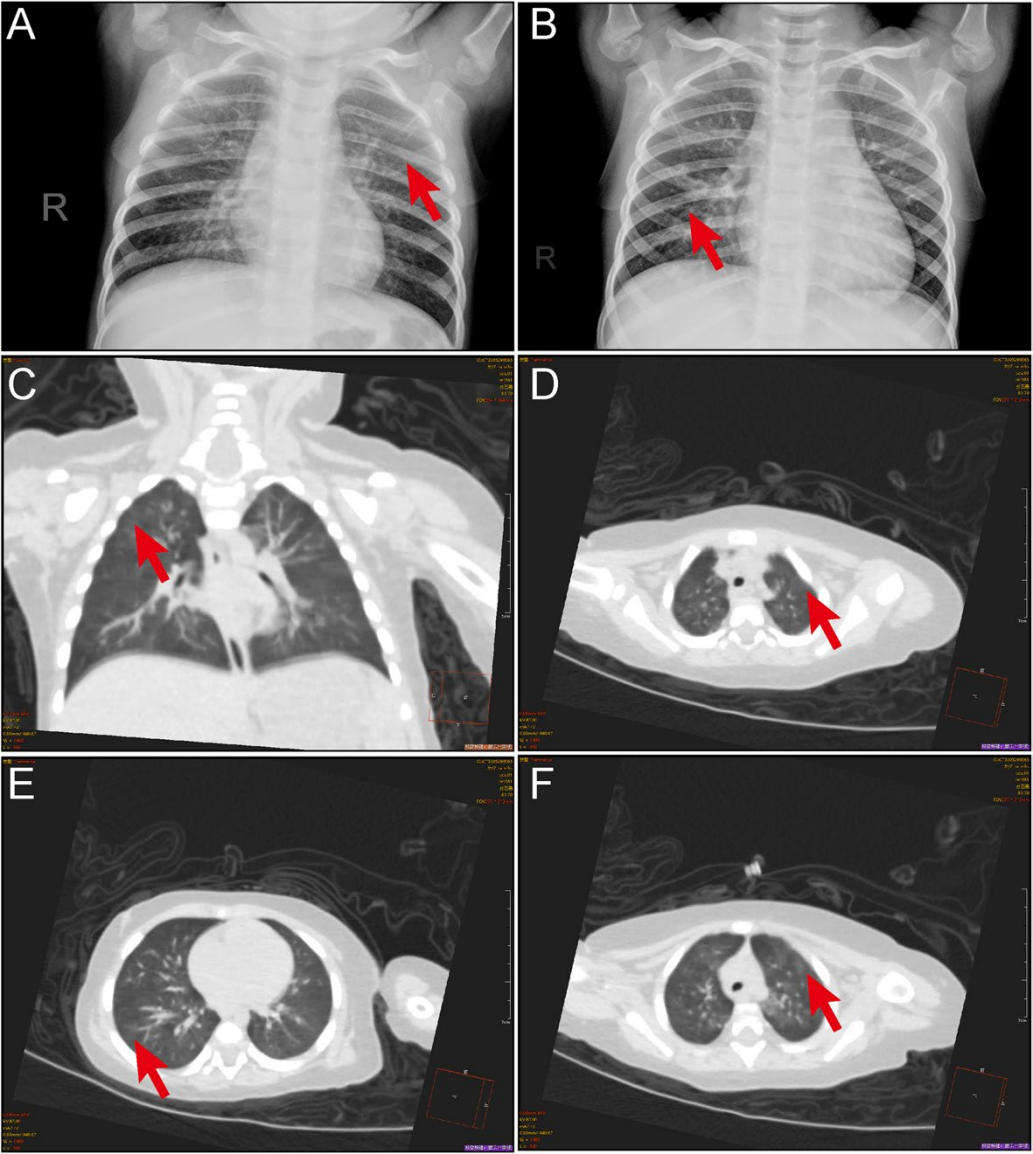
Pulmonary manifestations of HMPV infection. A CT scan of 1-year-old girl showing multiple ground-glass opacities in both lungs (arrow). B Chest radiograph of a 4-year-old girl displaying multiple ground-glass opacities (arrow). C-F Chest CT scans from a 7-month-old girl showing focal ground-glass opacities and stripes coexisting in the lung (arrows)
